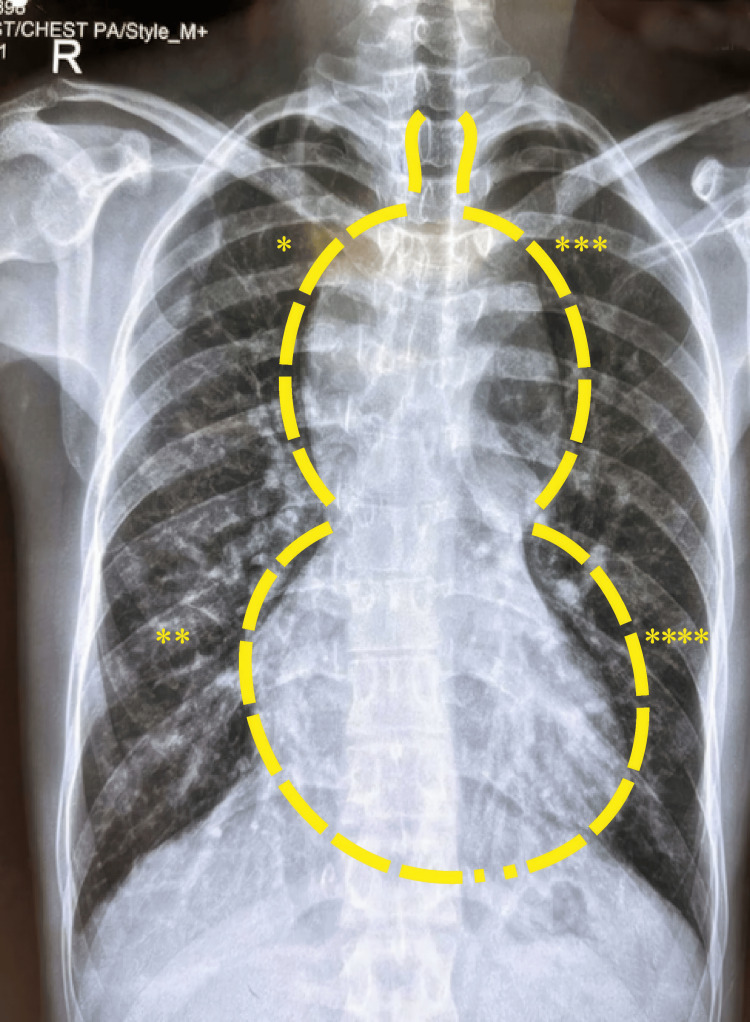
Preoperative thoracic X-ray of TAPVC. *: Dilated superior vena cava; : Right heart border; *: Dilated vertical vein; : Left heart border. TAPVC: Total anomalous pulmonary venous connection. Image credit: Dr. Sachin Talwar
